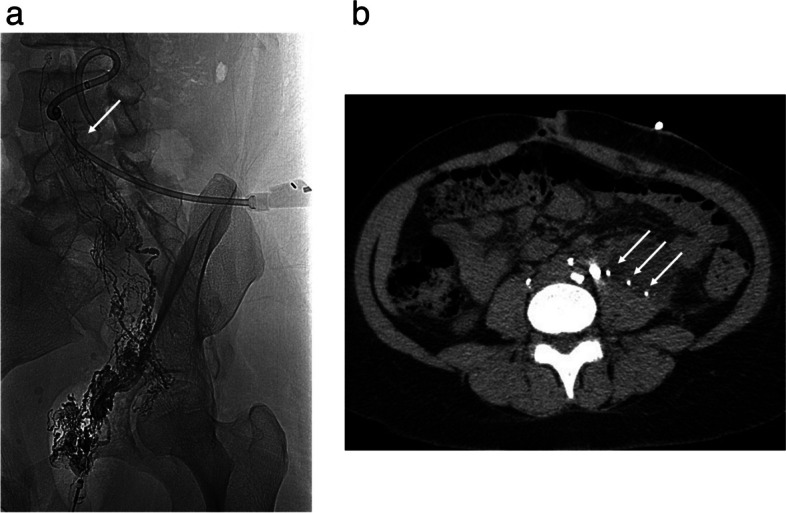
a, b Transnodal lymphangiography in a retroperitoneal lymphocele showing Lipiodol leak (arrow) (a). Computed tomography depicting Lipiodol droplets inside the lymphocele (multiple arrows) (b)
